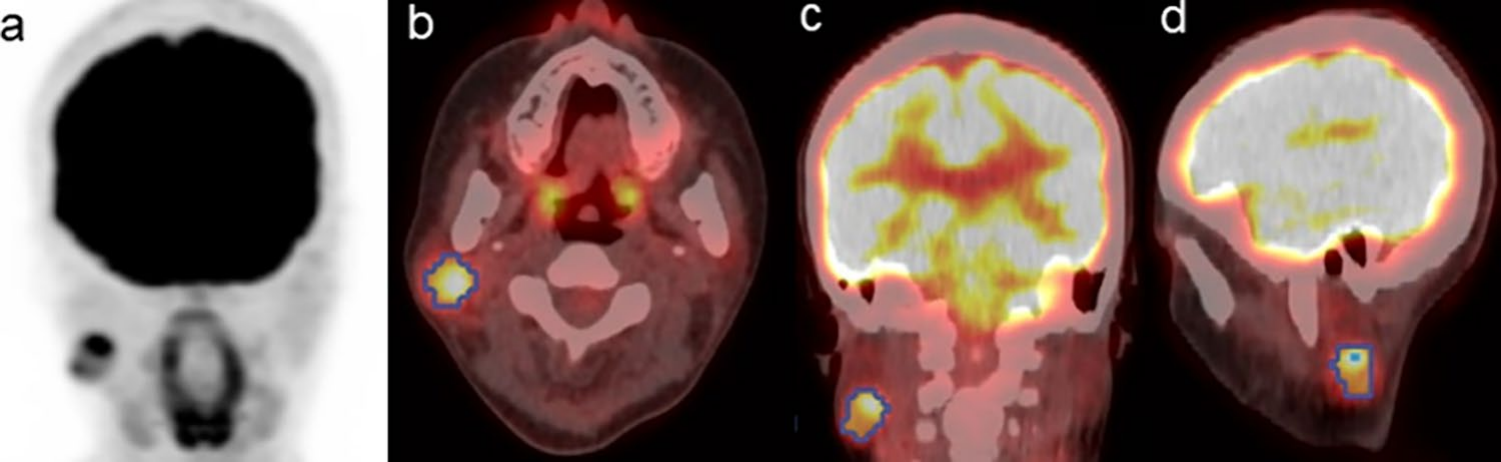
A 33-year-old female patient with a benign right parotid gland tumor (pleomorphic adenoma). Pretreatment 2-deoxy-2-[^18^F]fluoro-d-glucose ([^18^F]-FDG) positron emission tomography/computed tomography scan images [MIP (a), transaxial (b), coronal (c), and sagittal (d)] revealed focal [^18^F]-FDG uptake in the primary lesion. The blue line represents the border of the volume of interest. The calculated probability score for predicting malignancy (positive ≥ 0.5) was 0.38 in the DL-based ensemble model. Thus, the DL-based ensemble model can predict benign parotid gland disease in this case
